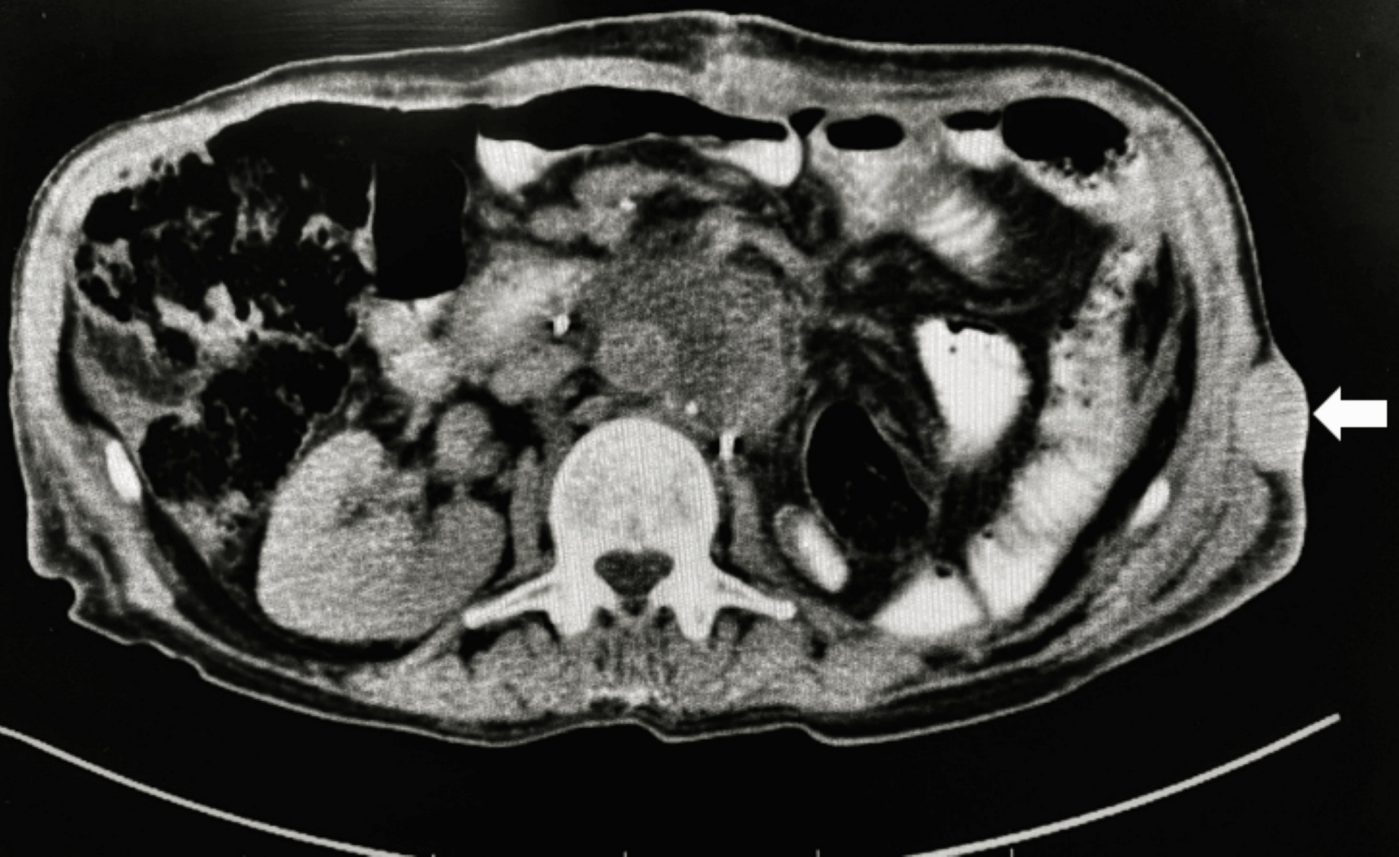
Follow-up enhanced CT scan of the abdomen and pelvis reveals a subcutaneous, homogeneously enhancing, rounded mass.
